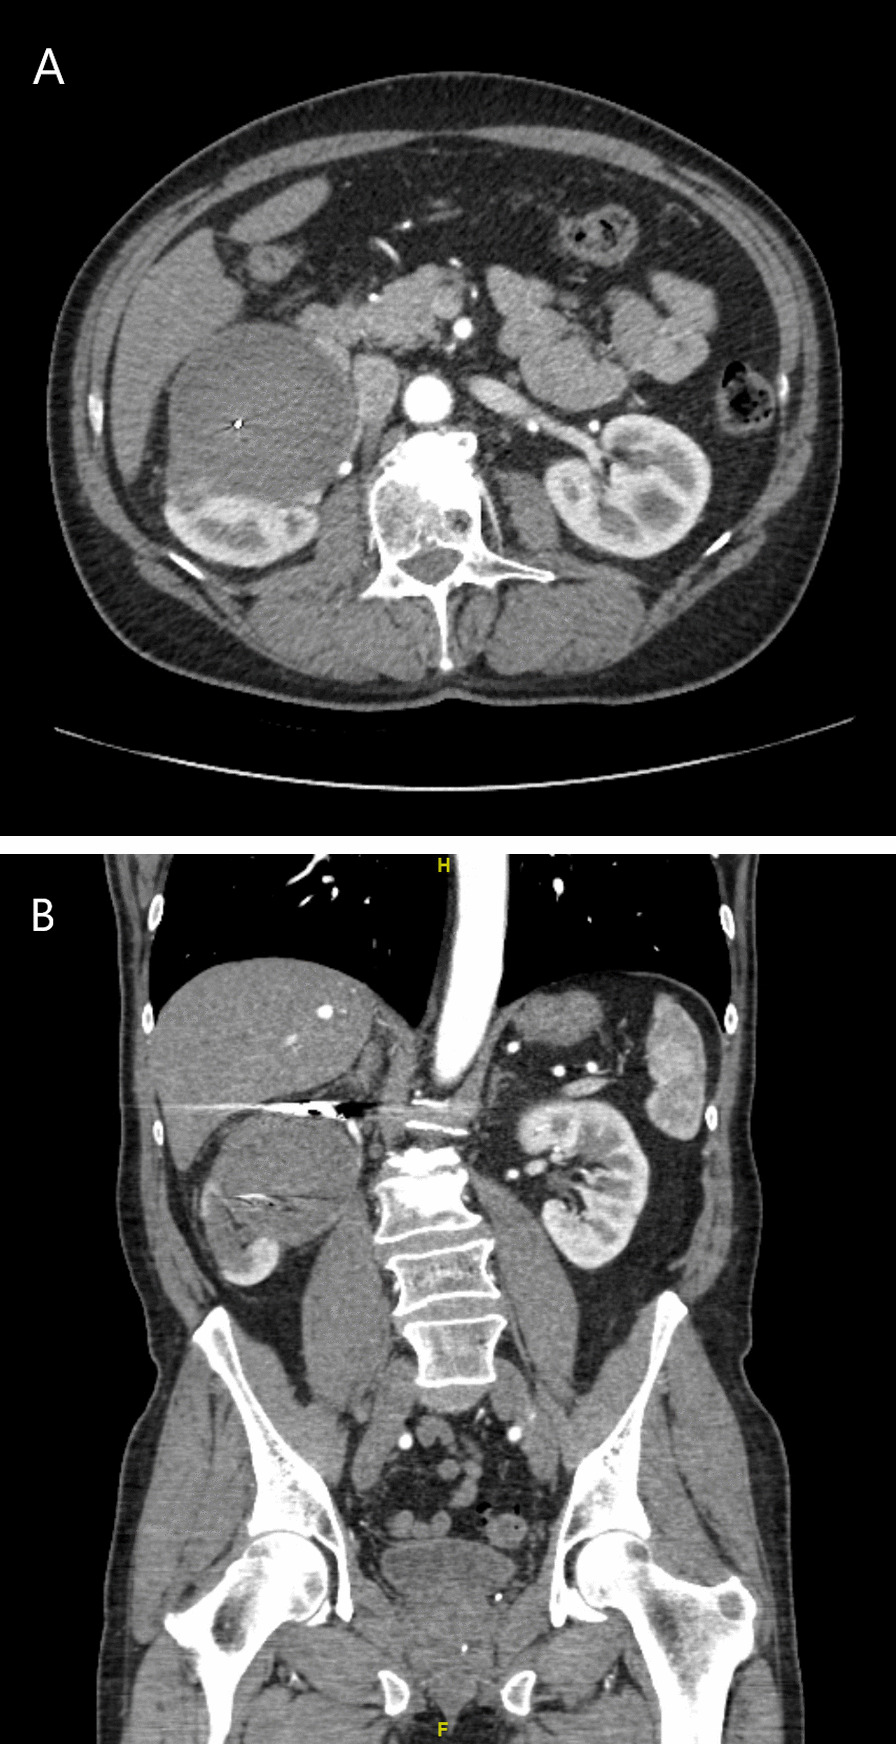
CTA (a, b) performed 3 months after angioembolization showed stable size of aneurysm sac. There was interval atrophy of right renal parenchyma due to renal infarct
